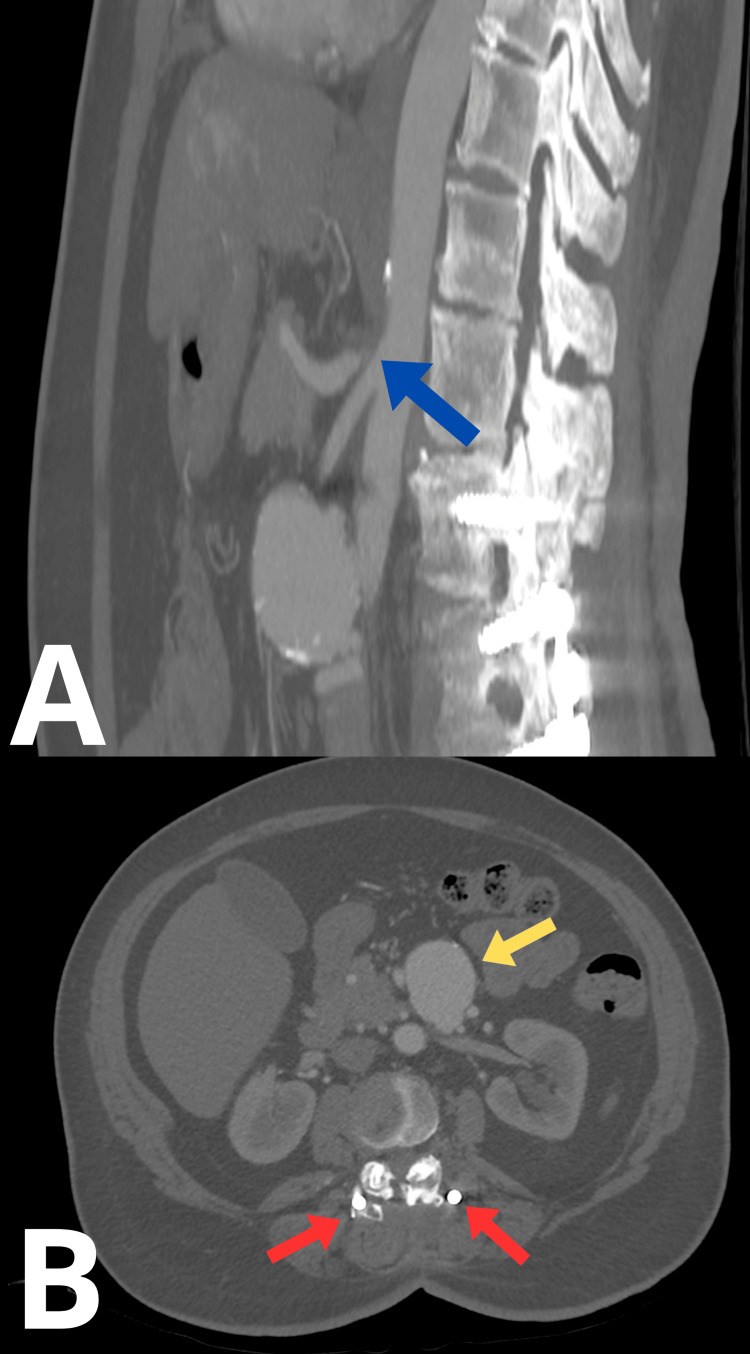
Initial CT scan. (A) Sagittal plane. (B) Transverse plane. The blue arrow indicates the compressed celiac trunk. The yellow arrow indicates the superior mesenteric artery aneurysm. The red arrow indicates spinal screws.
